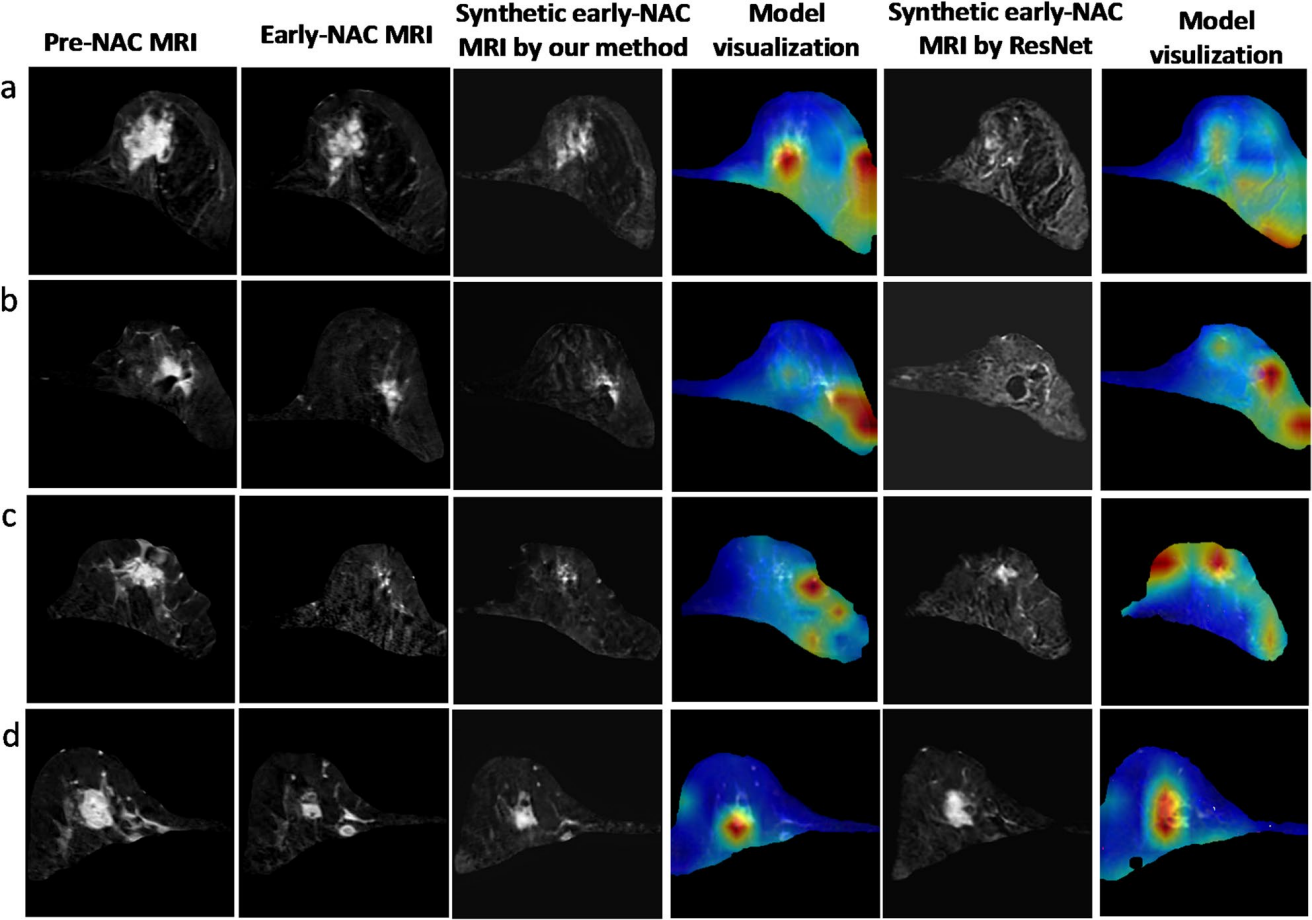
Model visualization examples for joint image generation and neoadjuvant chemotherapy (NAC) response prediction. From left to right, the columns display the preoperative MRI, the real early-NAC MRI, the synthetic early-NAC MRI images generated by the proposed method, the visualization of the proposed model, the synthetic early-NAC images created by a standard GAN model, and the corresponding class activation map for comparison. A A 50-year-old female patient exhibiting a non-pathological complete response (non-pCR) to NAC. B A 40-year-old female patient demonstrating a non-pCR following NAC. C A 61-year-old female patient who achieved a pCR. D A 49-year-old female patient who also achieved a pCR subsequent to NAC treatment
